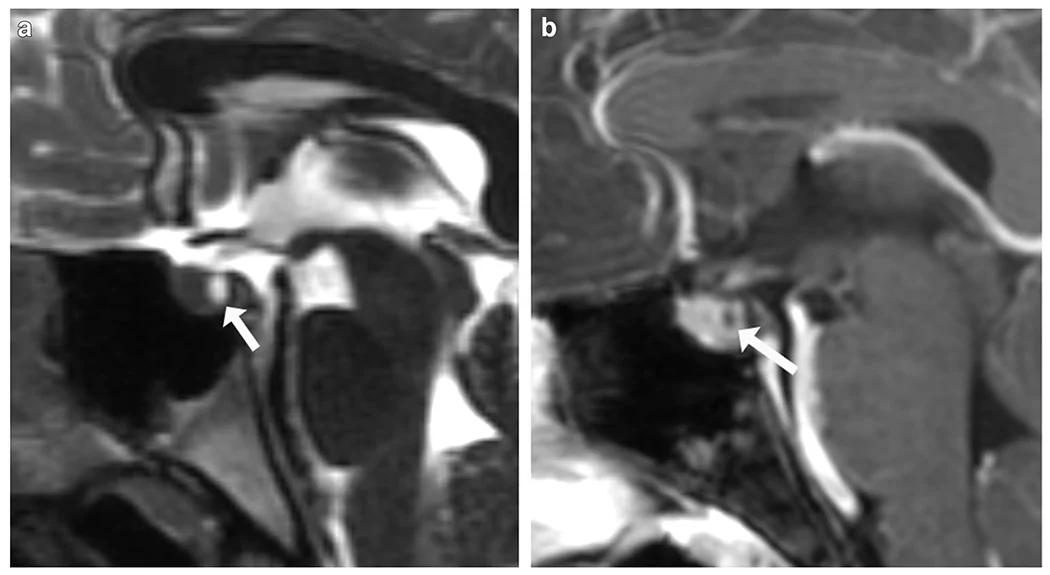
Rathke cleft cyst incidentally identified on a brain magnetic resonance imaging performed on a 16-year-old female with headache. a Sagittal T2-weighted image and (b) sagittal post-contrast T1-weighted image show a small rounded region of hyperintense T2-weighted signal (*arrow*) and non-enhancing isointense T1-weighted signal (*arrow*) located between the anterior pituitary and the posterior pituitary bright spot, consistent with a small Rathke cleft cyst
